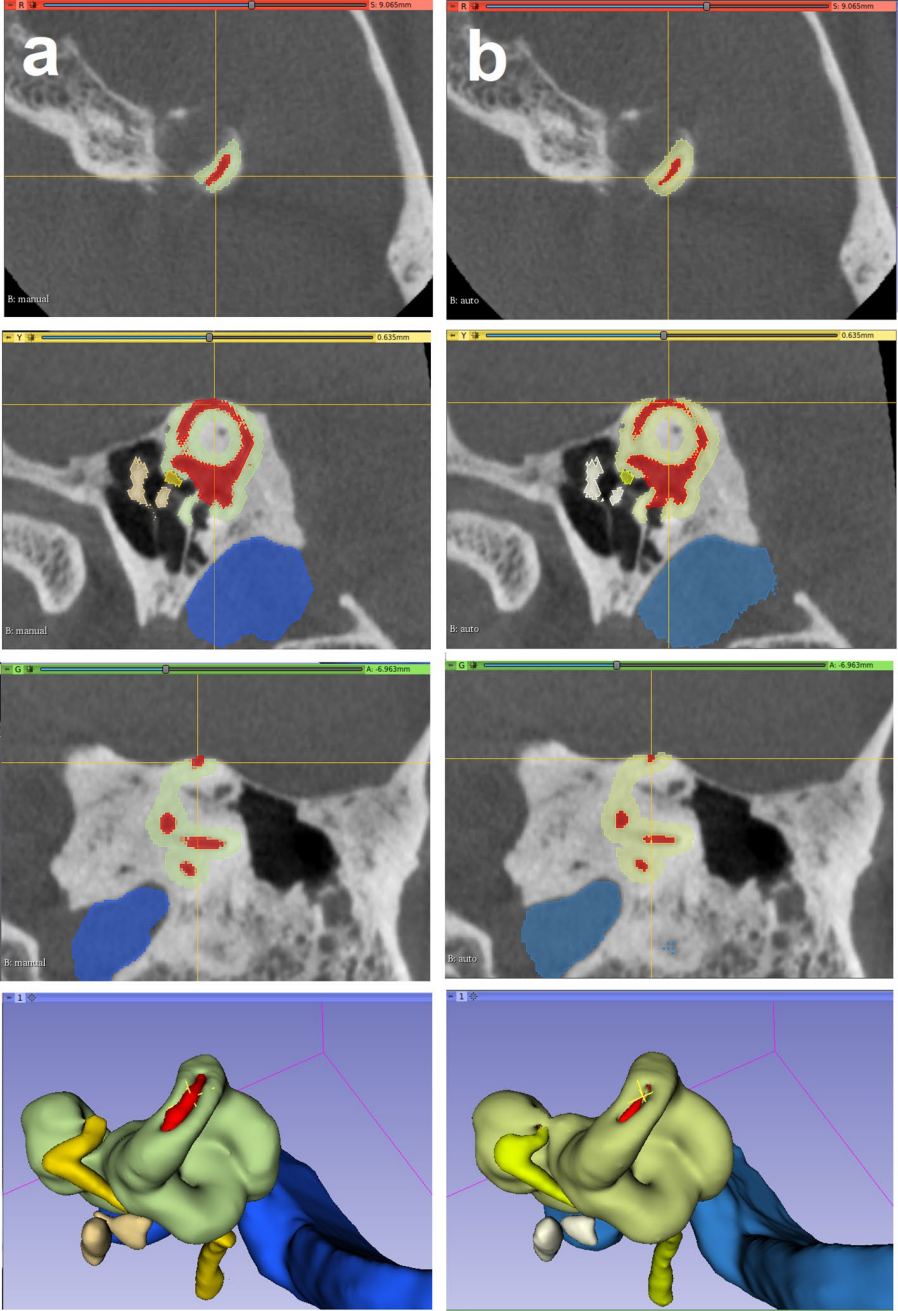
Superior semicircular canal dehiscence as demonstrated from manual (a) and auto-segmented (b) CT dataset seen in 3D Slicer. Note the lack of bone covering the balance canal (at the crosshair). Understanding the location and size of such a defect can facilitate surgical planning. Inner ear (red), ossicles (ivory); facial nerve (yellow), sigmoid sinus (blue) and otic capsule (green).
